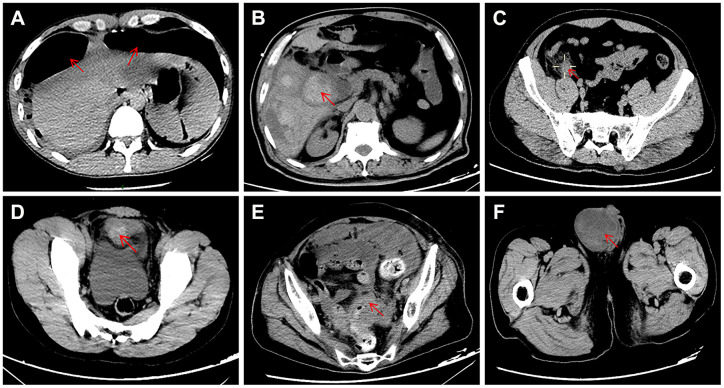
Typical appearance of abdominal CT showing the causes of acute abdomen in the present study. (A) duodenal perforation accompanied by free intraperitoneal gas; (B) gangrenous cholecystitis; (C) acute appendicitis; (D) bladder rupture; (E) intestinal obstruction caused by carcinomas in the rectosigmoid junction; (F) intestinal obstruction caused by inguinal incarcerated hernia.
